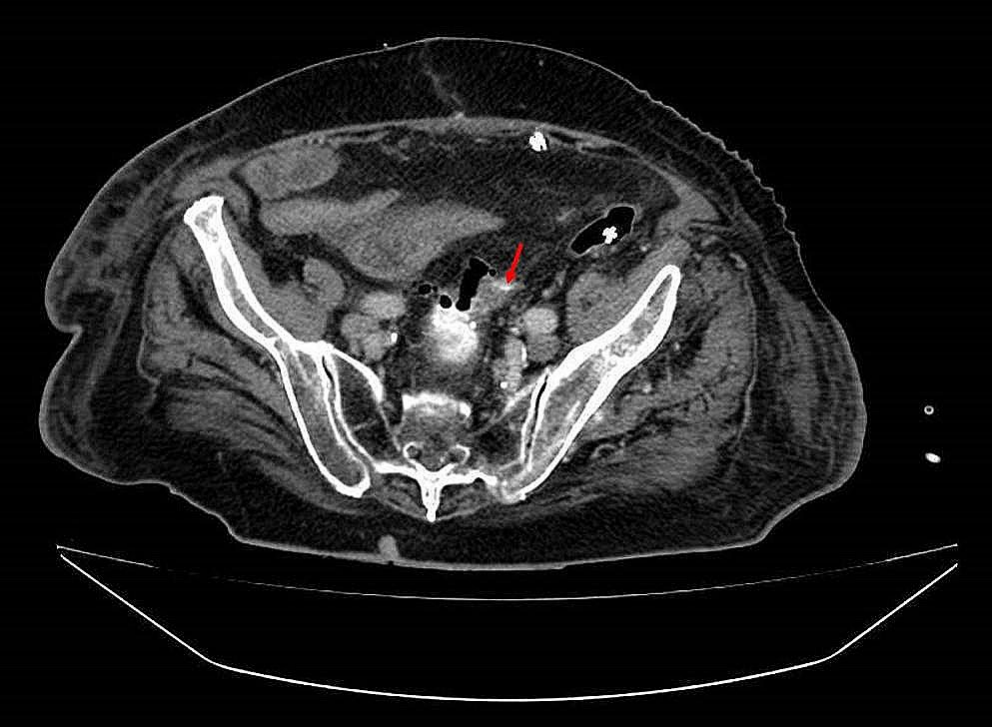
Abdominal CT of AL with rectal contrast medium leakage (red arrow)
